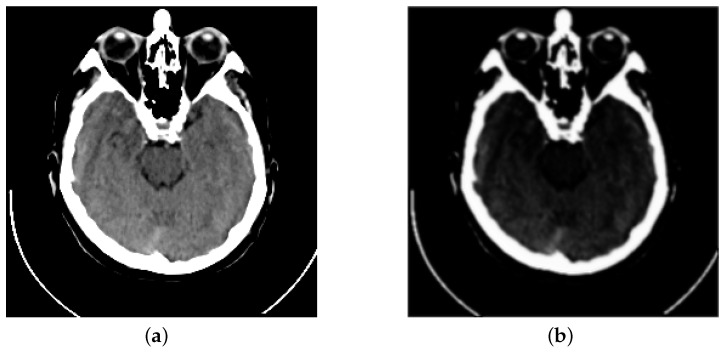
Example of a CT image and its visual saliency map. (a) The source CT image of “Acute stroke speech arrest-3”. (b) The visual saliency map of this CT image.
